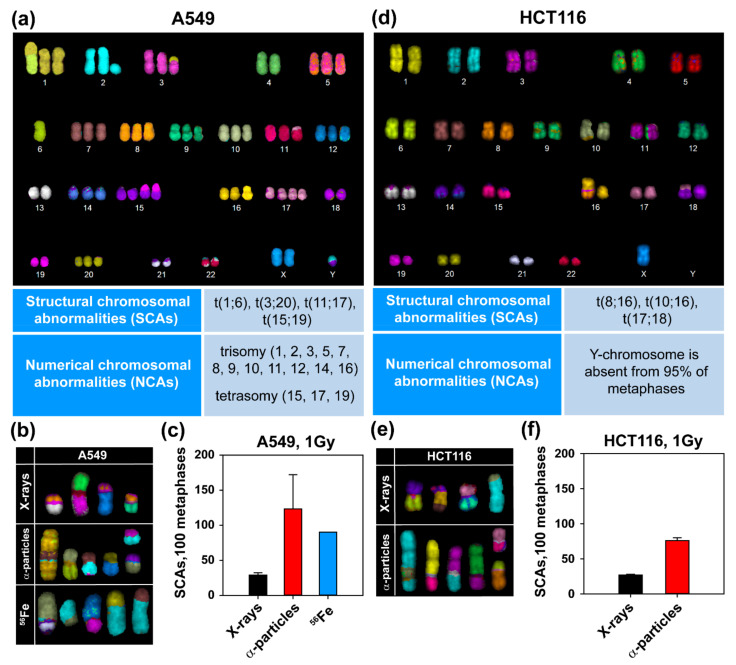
Increased formation of SCAs after exposure to high-LET IR. (a) mFISH karyotype map of A549 cells with annotation of constitutive SCAs and NCAs. (b) Representative SCAs in A549 cells, exposed to different forms of IR. (c) Quantitative analysis of SCAs in A549 cells exposed to X-rays, α-particles and ^56^Fe ions. (d) As in (a), but for HCT116 cells. (e) As in (b), but for HCT116 cells. (f) As in (c), but for HCT116 cells. At least 50 metaphases were scored for each condition. Data represent the mean ± SD from three experiments. Results for ^56^Fe ions are from a single experiment.
